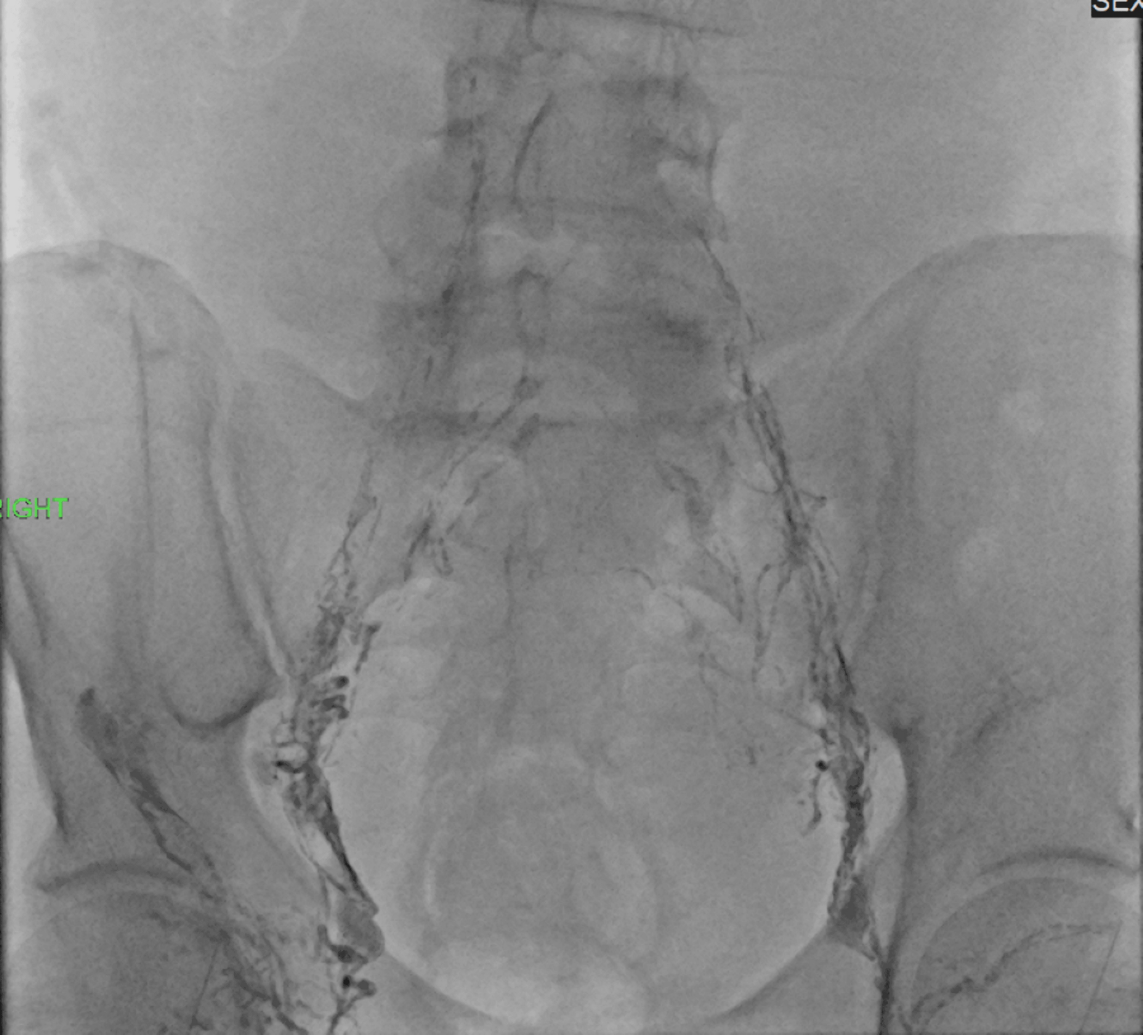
After ultrasound-guided puncture of several bilateral inguinal lymph nodes with 25G needles, lipiodol was injected, and pelvic lymphangiography was obtained
